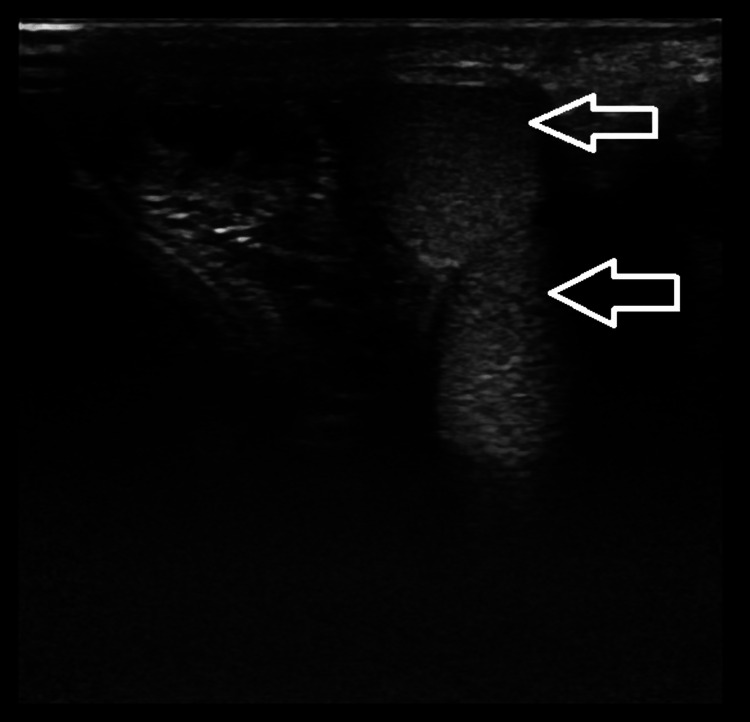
Gray-scale ultrasound image showing one testis in the left scrotal sac with another testis (white arrows) at the root of the left scrotum.
